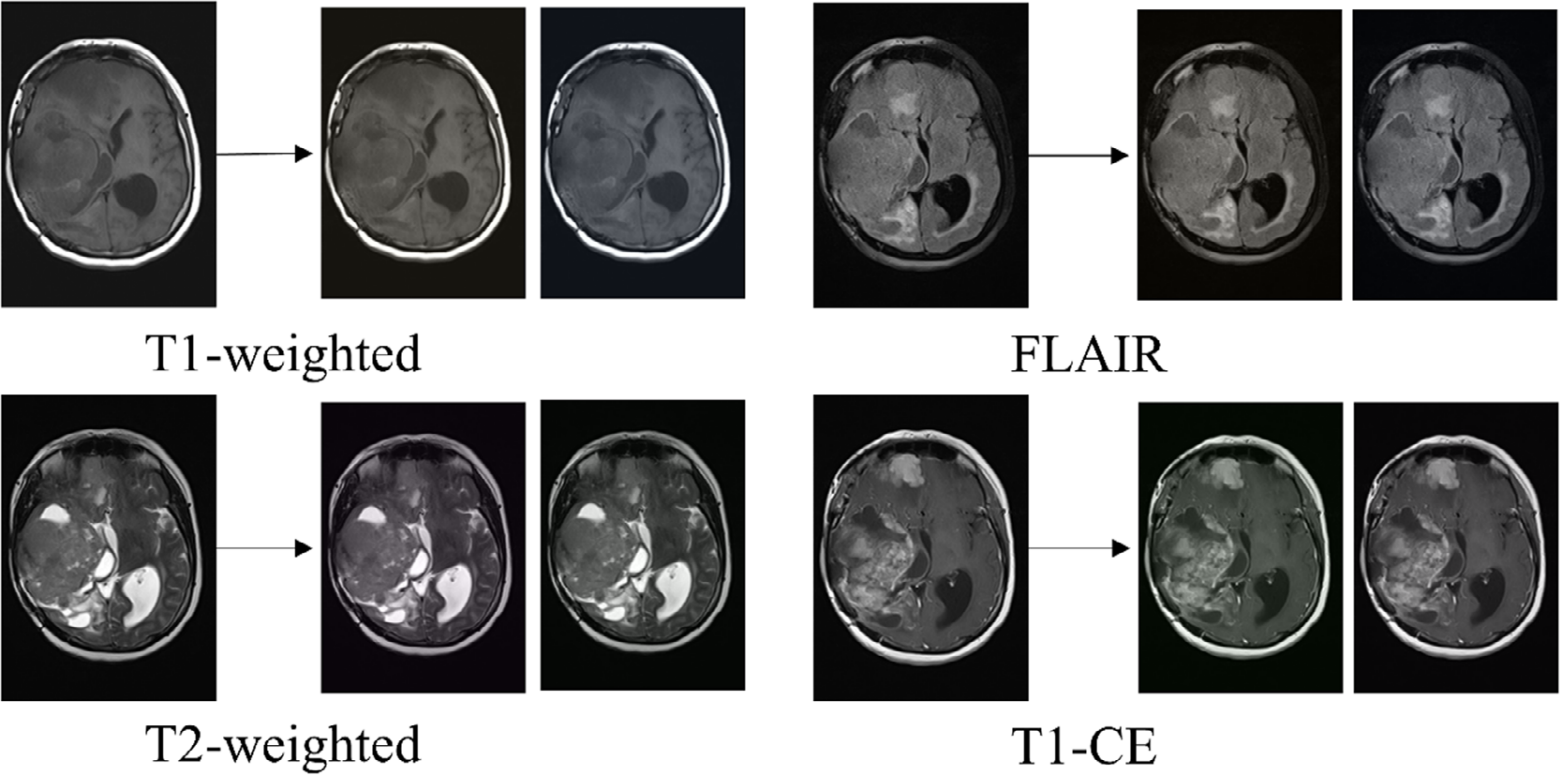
Samples of meningioma MR images and two artificially generated images with fancy PCA (images on the left and right of the arrows are original and augmented images, respectively).
